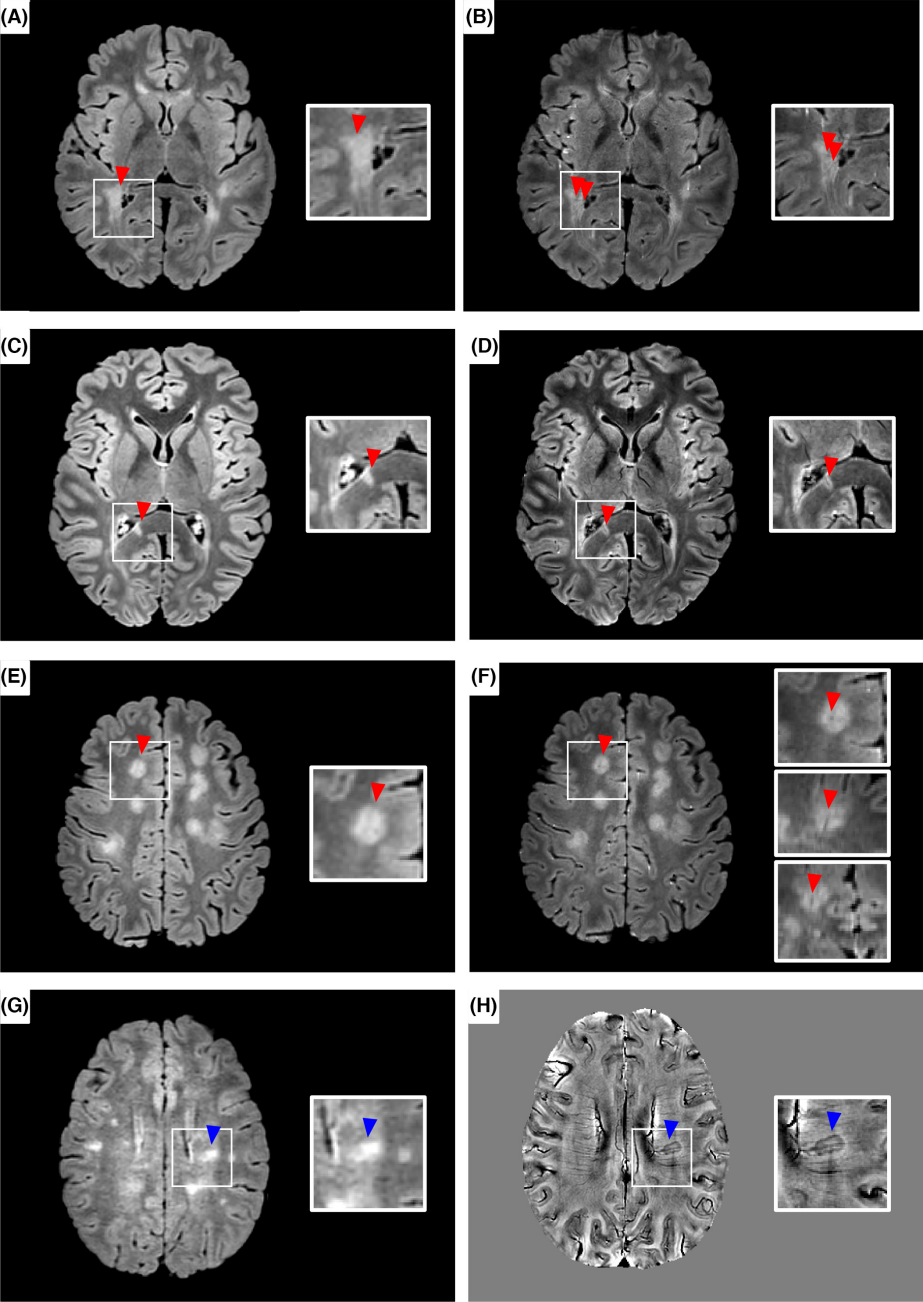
White matter lesions with central vein sign and paramagnetic rim in patients with pediatric multiple sclerosis. Axial fluid‐attenuated inversion recovery (FLAIR) sequence showing a confluent lesion (A) with multiple veins within its context on FLAIR* (B). Axial (C,E) FLAIR sequences showing brain T2‐hyperitense white matter lesions that, on FLAIR* (D,F) demonstrate a central vein sign (red triangles). In (F) the central vein sign is visible in the three plains (axial, sagittal, and coronal). Axial FLAIR sequence (G) showing a brain T2‐hyperitense white matter lesion that, on phase image (H), demonstrates a paramagnetic rim (blue triangle). See text for further details.
